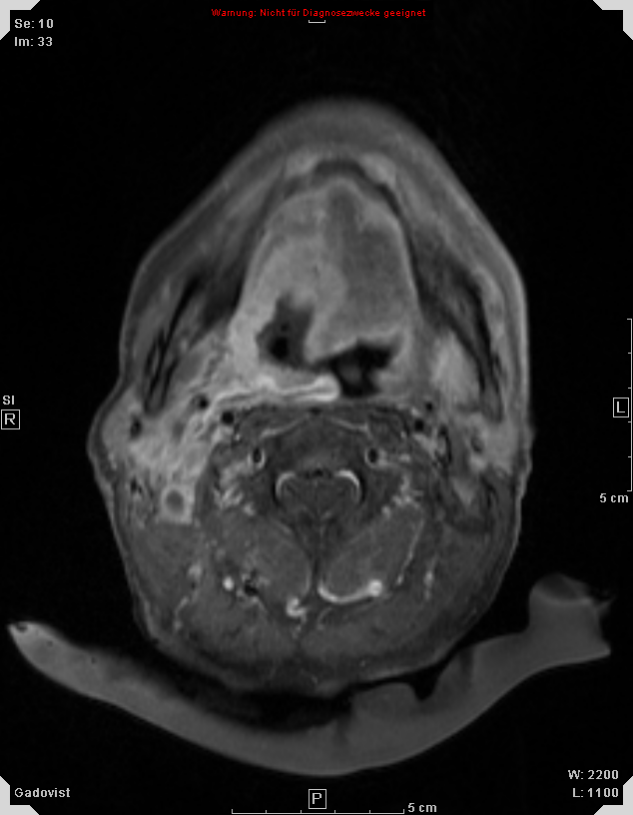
50 y old patient with oro-/hypopharyngeal carcinoma; planning MRI.
